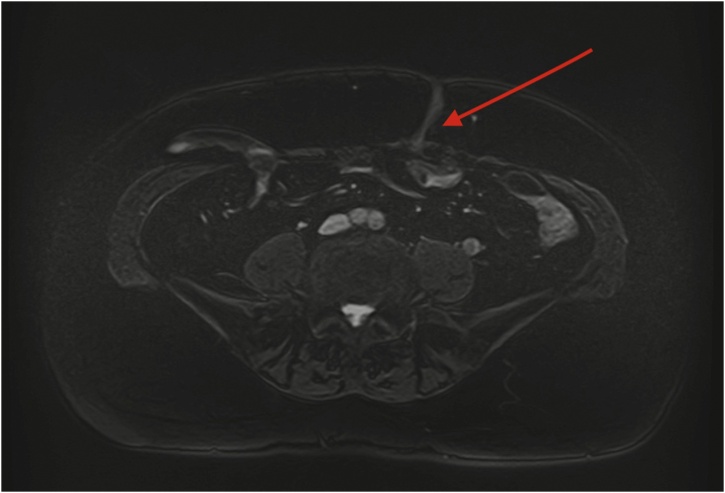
MRI image of the ECF in the axial plane.
